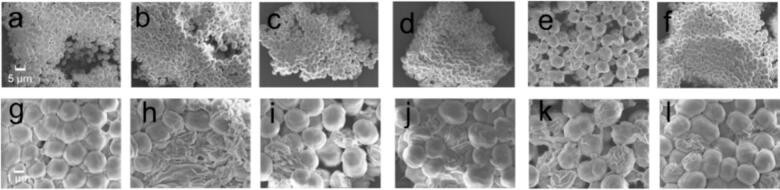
SEM images of algae before and after sonication at two different magnifications: 10 k (subfigures a to f) and 30 k (subfigures g to l). These images depict the algae at various stages: (a, g) prior to treatment, (b, h) immediately following a 20-minute ultrasound session, (c, i) at 2.5 days post-treatment, (d, j) after the second ultrasound application, (e, k) at 3 days, and (f, l) at 5 days.
